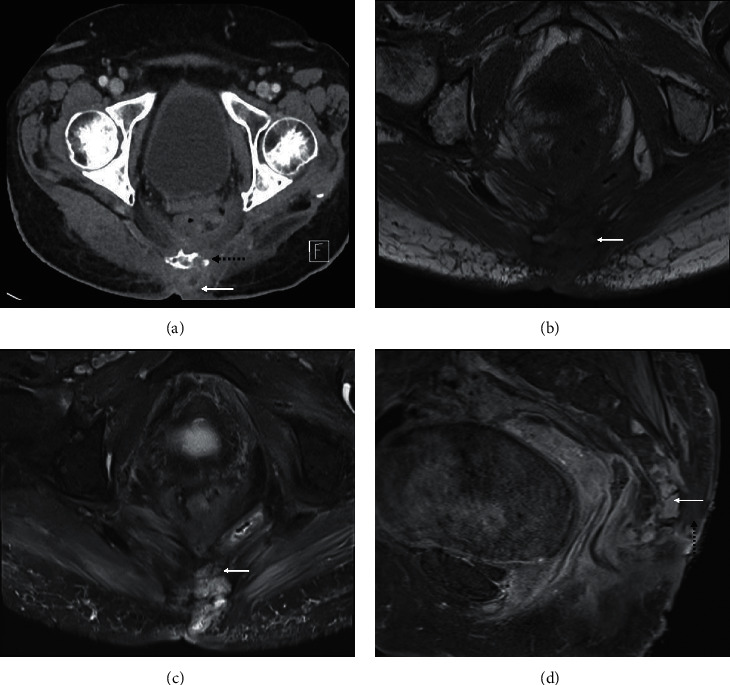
Osteomyelitis with an ulcer tract arising from a necrotic, infected pressure sore. Axial CT pelvis with intravenous contrast (a) of a 78-year-old bedbound female worked up for sepsis secondary to an infected pressure ulcer showed the ulcer extending to the coccyx in the left parasagittal region (arrow), with bone erosion (dotted line). The affected bone is hypointense on the axial T1 (b) and hyperintense on fluid-sensitive, PDFS (proton density with fat saturation, (c)) sequences. Sagittal T1FS postcontrast image (d) shows enhancement of the coccyx (arrow) and sacrococcygeal joint confirming the diagnosis. The adjacent deep subcutaneous region of nonenhancement is compatible with the area of necrosis (dotted arrow).
